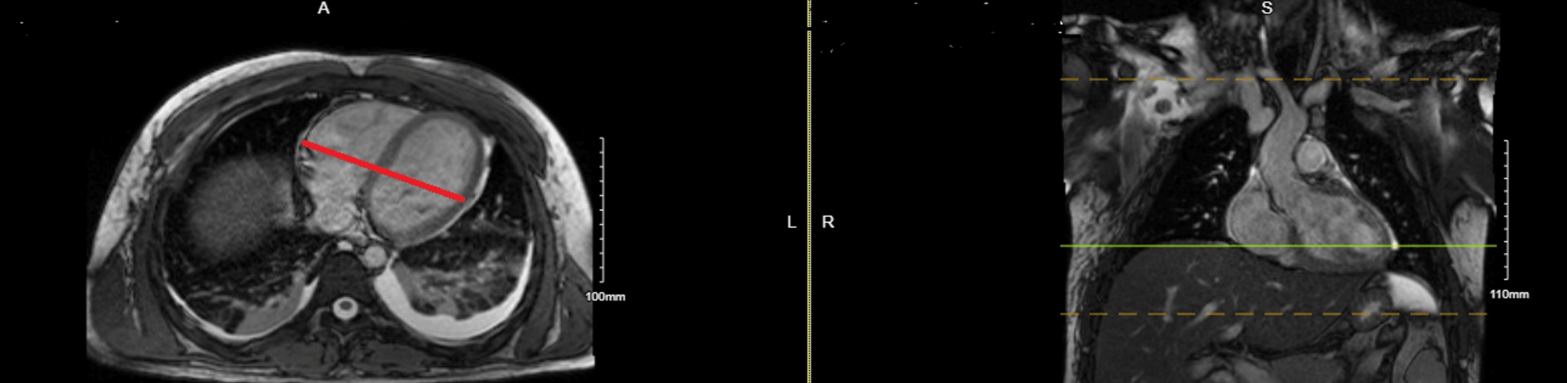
MRI taken after the resolution of the patient’s myocarditis. The image reveals normal left ventricular size, wall thickness, as well as absence of delayed enhancement to suggest infiltrative process.
